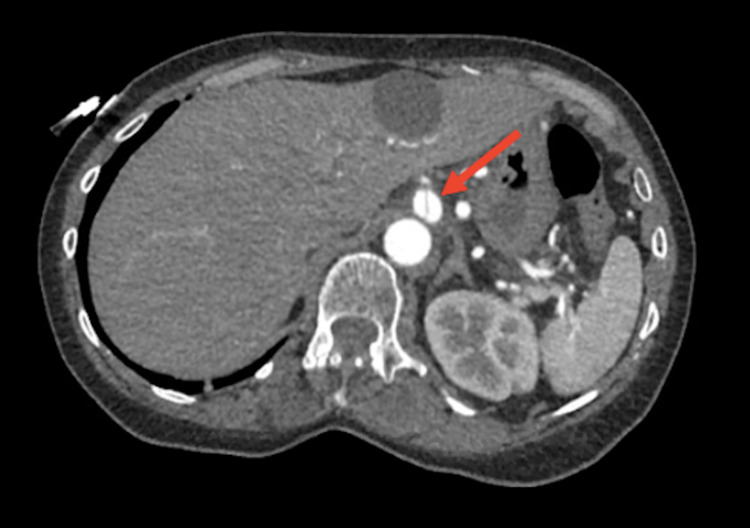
Axial CTA demonstrating celiac artery dissection (arrow) CTA: computed tomography angiography.
